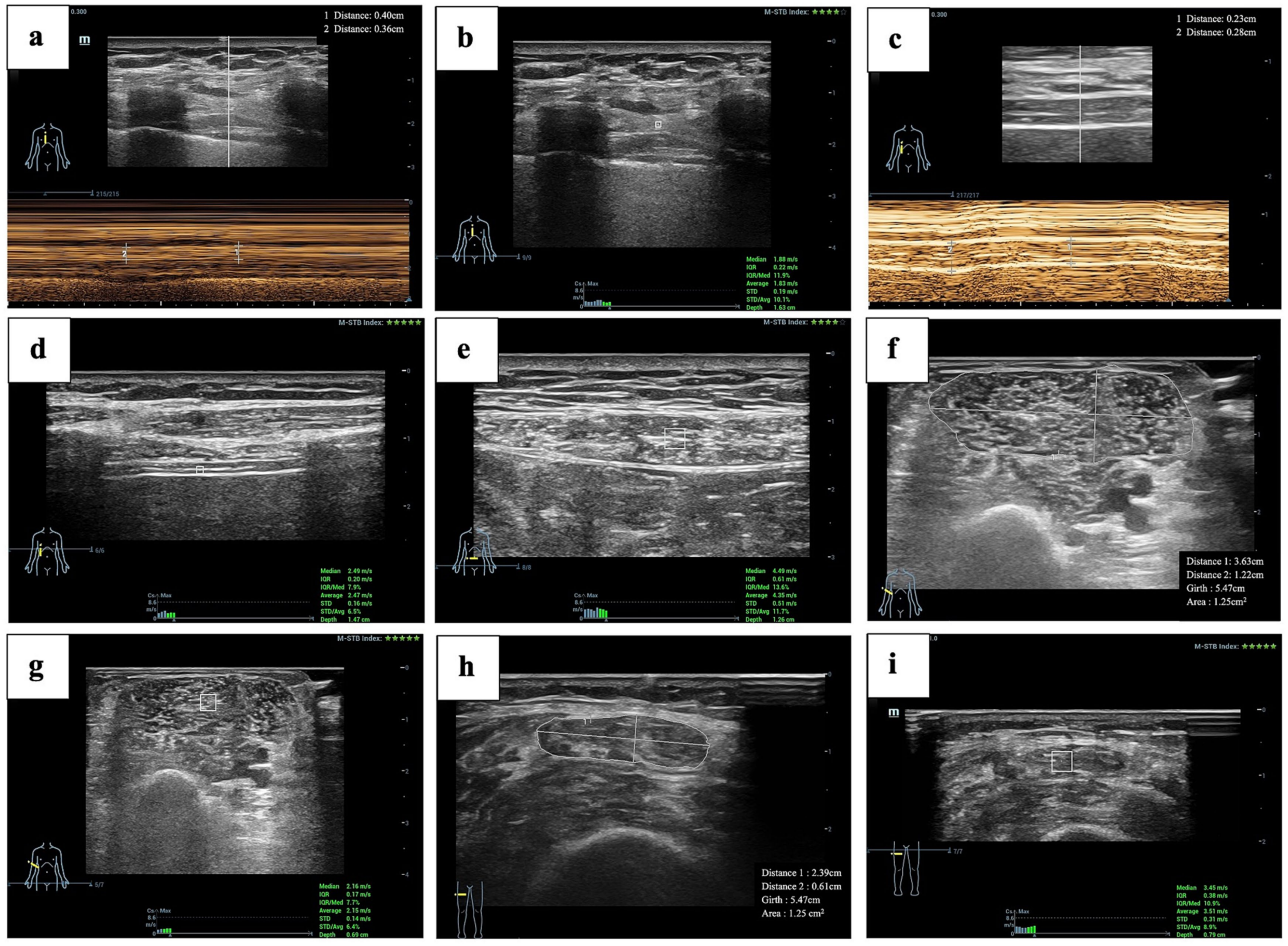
Clinical examples of multi-site multi-parameter ultrasound assessment. Clinical case of a 72-year-old male with COPD undergoing sarcopenia screening using multi-site and multi-parameter ultrasound. The patient has a history of over 30 years of COPD, a BMI of 19.03, calf circumference of 35 cm, and a right-hand dominant grip strength of 18.1 kg. Following ultrasound examination and clinical assessment, the patient was considered to have concomitant sarcopenia. Subsequently, a nutritionist devised a personalized intervention program. This program comprised a high-protein diet, probiotic supplements, and an exercise plan. (a) M-mode ultrasound measurement of intercostal muscle thickness at different phases of respiration. (b) Shear wave elastography (SWE) measurement of intercostal muscle shear wave velocity (SWV). (c) M-mode ultrasound measurement of diaphragm thickness at different phases of respiration. (d) SWE measurement of diaphragm shear wave velocity (D-SWV). (e) SWE measurement of rectus abdominis shear wave velocity (RA-SWV). (f) Cross-sectional area measurement of biceps brachii (BB-CSA). (g) SWE measurement of biceps brachii shear wave velocity (BB-SWV). (h) Cross-sectional area measurement of rectus femoris (RF-CSA). (i) SWE measurement of rectus femoris shear wave velocity (RF-SWV).
